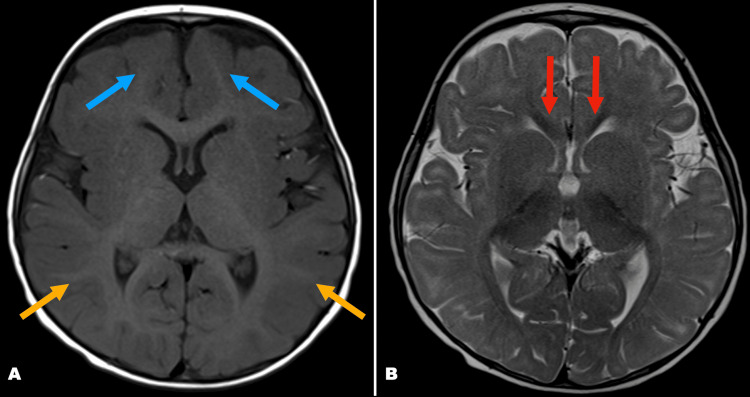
MRI findings indicating improved myelination Axial T1 (A) and T2 (B) at 16 months showing interval improvement in myelination of the corpus callosum (red arrows), parieto-occipital lobes (yellow arrows), and other brain white matter structures (blue arrows).
